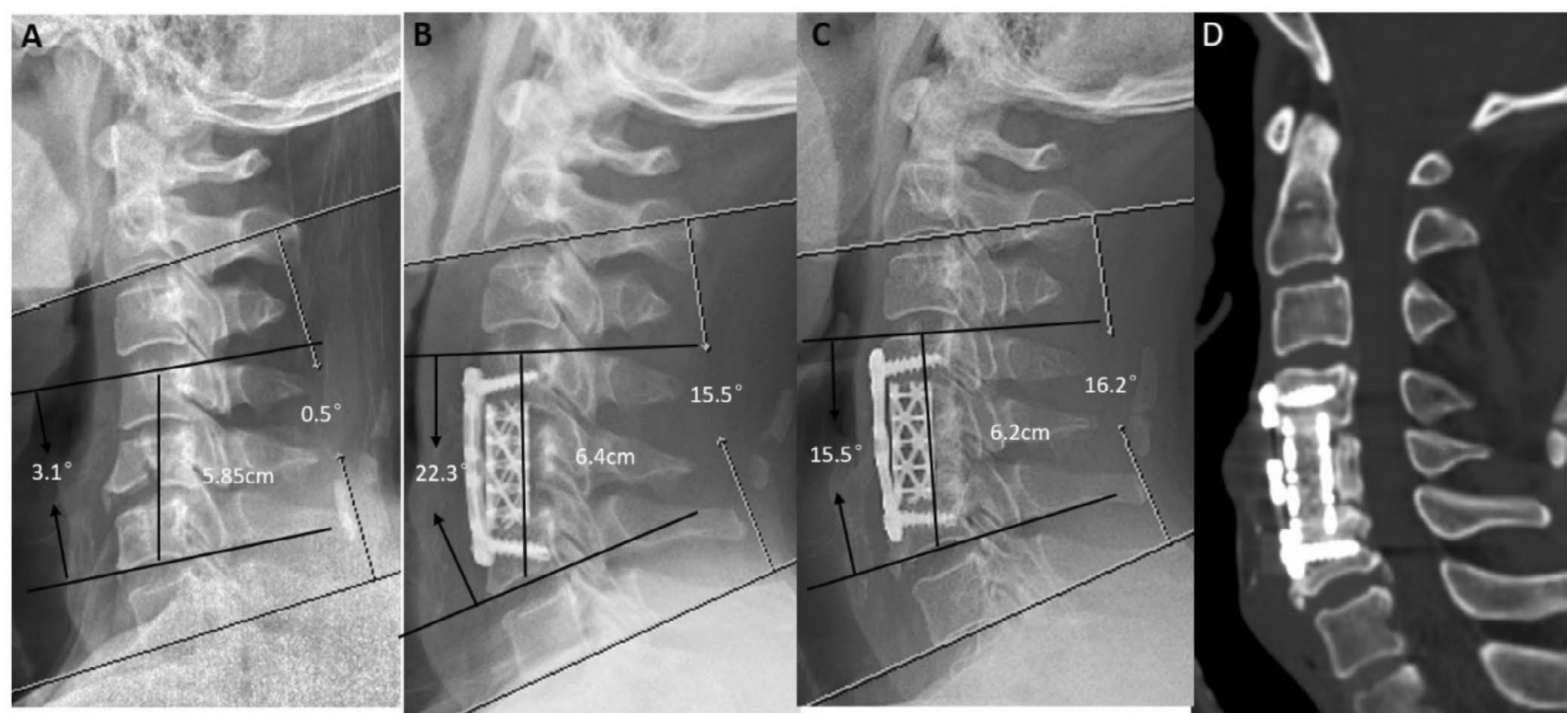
A 43‐year‐old male who underwent 1‐level corpectomy with a titanium mesh cage used for cervical reconstruction. The preoperative cervical X‐ray film (A) (showing a C5 burst fracture): 1 week postoperative lateral X‐ray (B) (C5 corpectomy and the titanium mesh cage used for reconstruction improved CL, SA, and IH). The radiographic films (C) and 3D‐CT (D) scan showing satisfying bony fusion at the 9‐year follow‐up with a little cage subsidence and partial bone resorption. CL, cervical lordosis; IH, intervertebral height; SA, segmental alignment.
